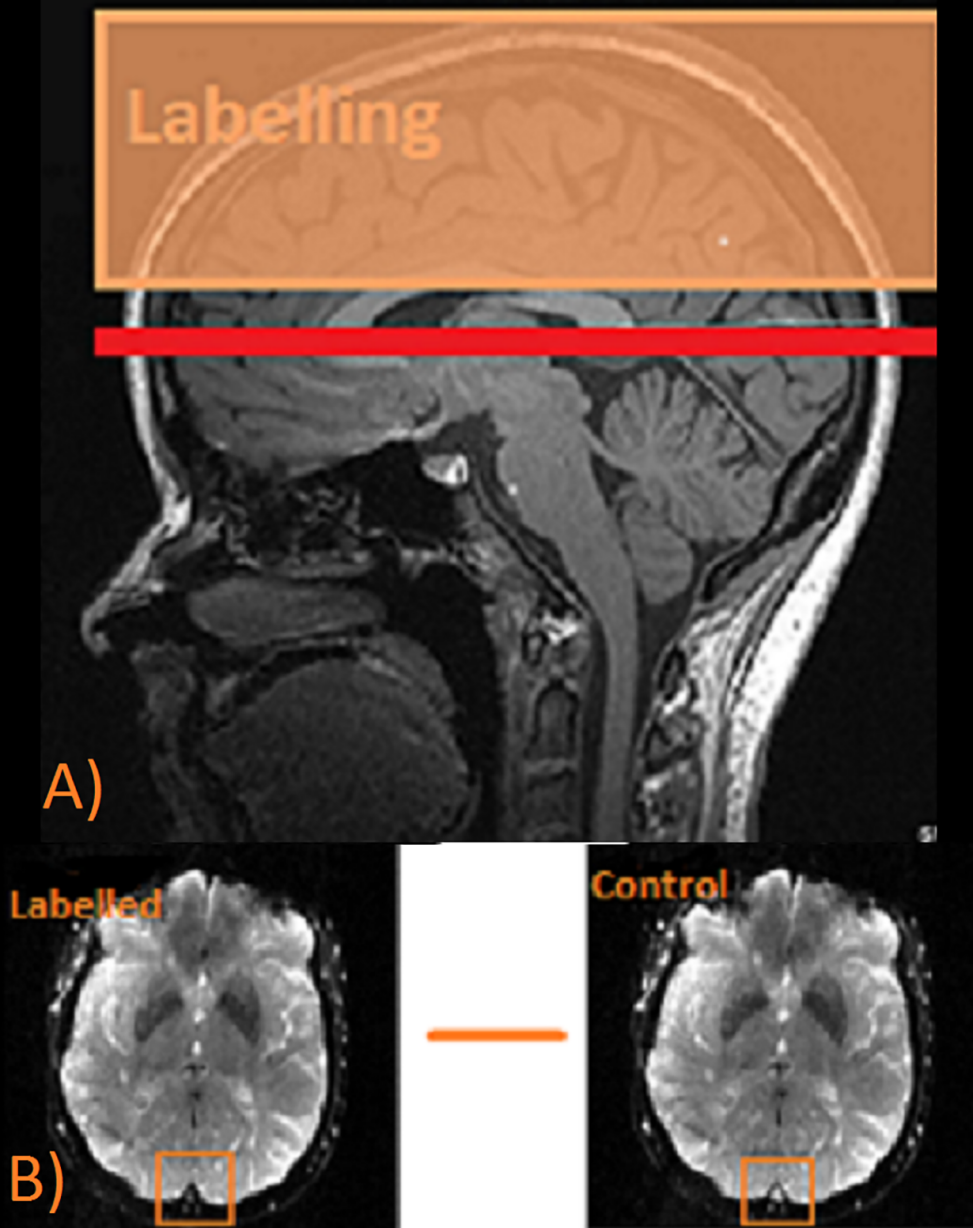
Quantification of the venous oxygenation using T2- relaxation-under- spin- tagging (TRUST) MRI A) The location of the labeling slab region (orange block) of the TRUST scan upstream from the imaging slice (red line). T2 blood signal was acquired from the superior sagittal sinus (SSS) B) Raw axial images of the control and labelled scans, SSS region is shown in the orange box. The label inverts the venous blood signal so it appears dark in the image.
